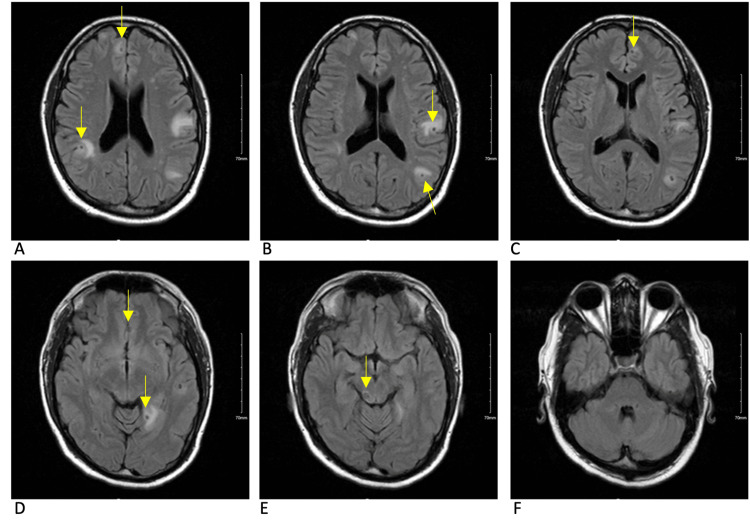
MRI brain T2 flair sequence 13 weeks after initial symptom onset Areas of inflammation that do not correlate to an obvious cyst in the T1 post-contrast sequence are seen in the left and right parietal lobes (panes A and B). The anterior right frontal lobe cyst has increased inflammation when compared to the prior scan (pane A). The anterior left frontal lobe cyst has resolved since the prior scan (pane B). The interhemispheric lesion (pane D) has minimal inflammation demonstrating the beginning of cyst degeneration. The left hippocampal lesion has significant inflammation associated with it (pane D). The right posterior midbrain lesion has slightly increased inflammation (pane E).
